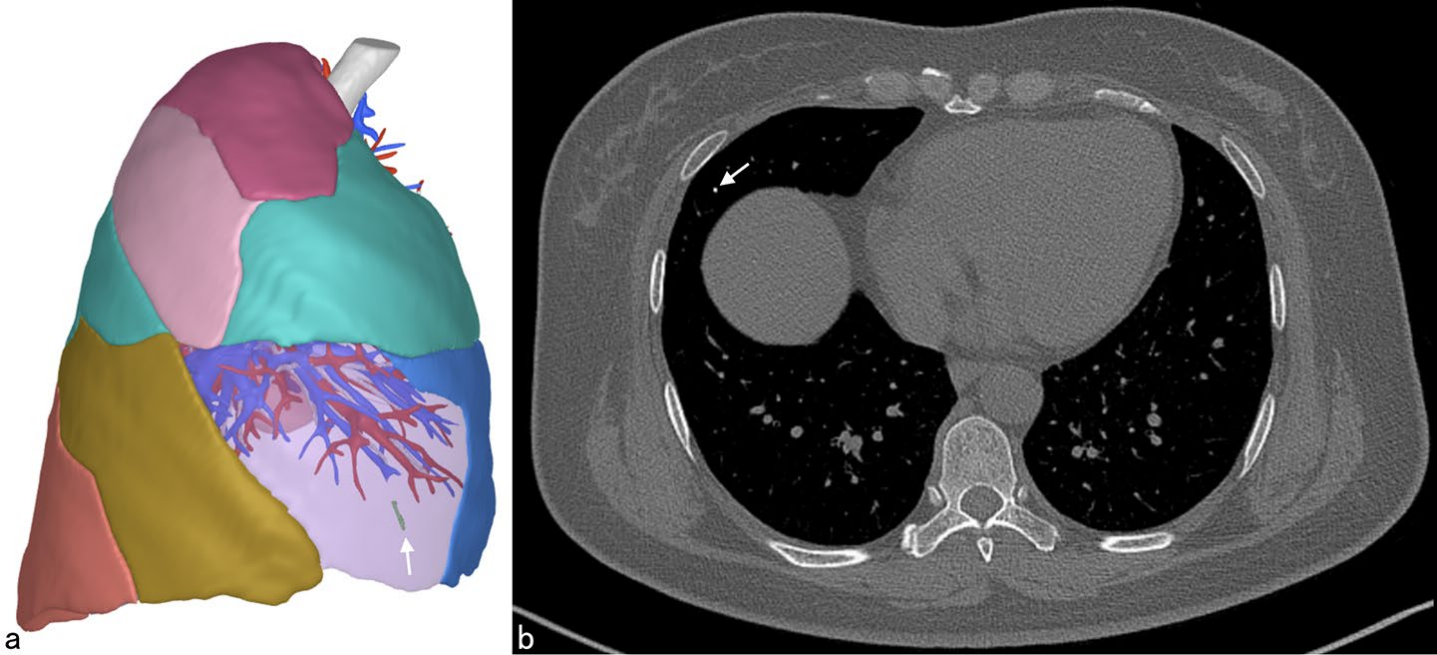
Preoperative imaging localization: (a) 3D reconstruction (white arrow); (b) CT scan (white arrow)
